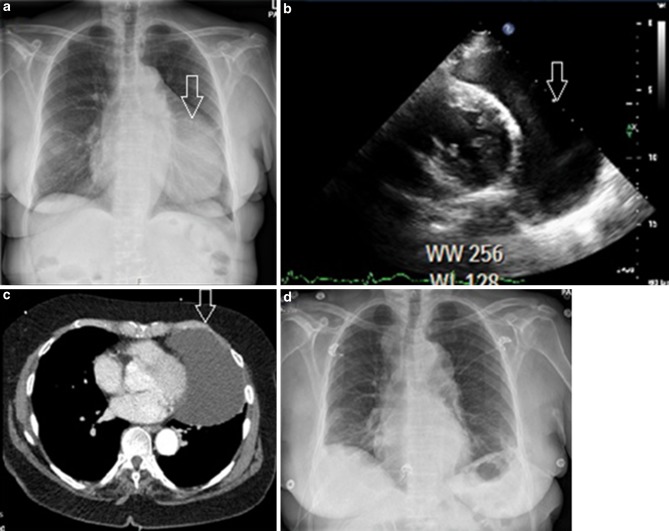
a Chest radiography showing an enlarged cardiac silhouette, b TTE showing the presence of an echo-lucent space adjacent to the left ventricle, c CT heart image showing a lobulated, low-signal-intensity, cystic mass within the pericardium to the left of the left heart border, d Chest radiography showing a normal heart contour after surgical removal of the pericardial cyst (*CT* computed tomography, *TTE* transthoracic echocardiography)
